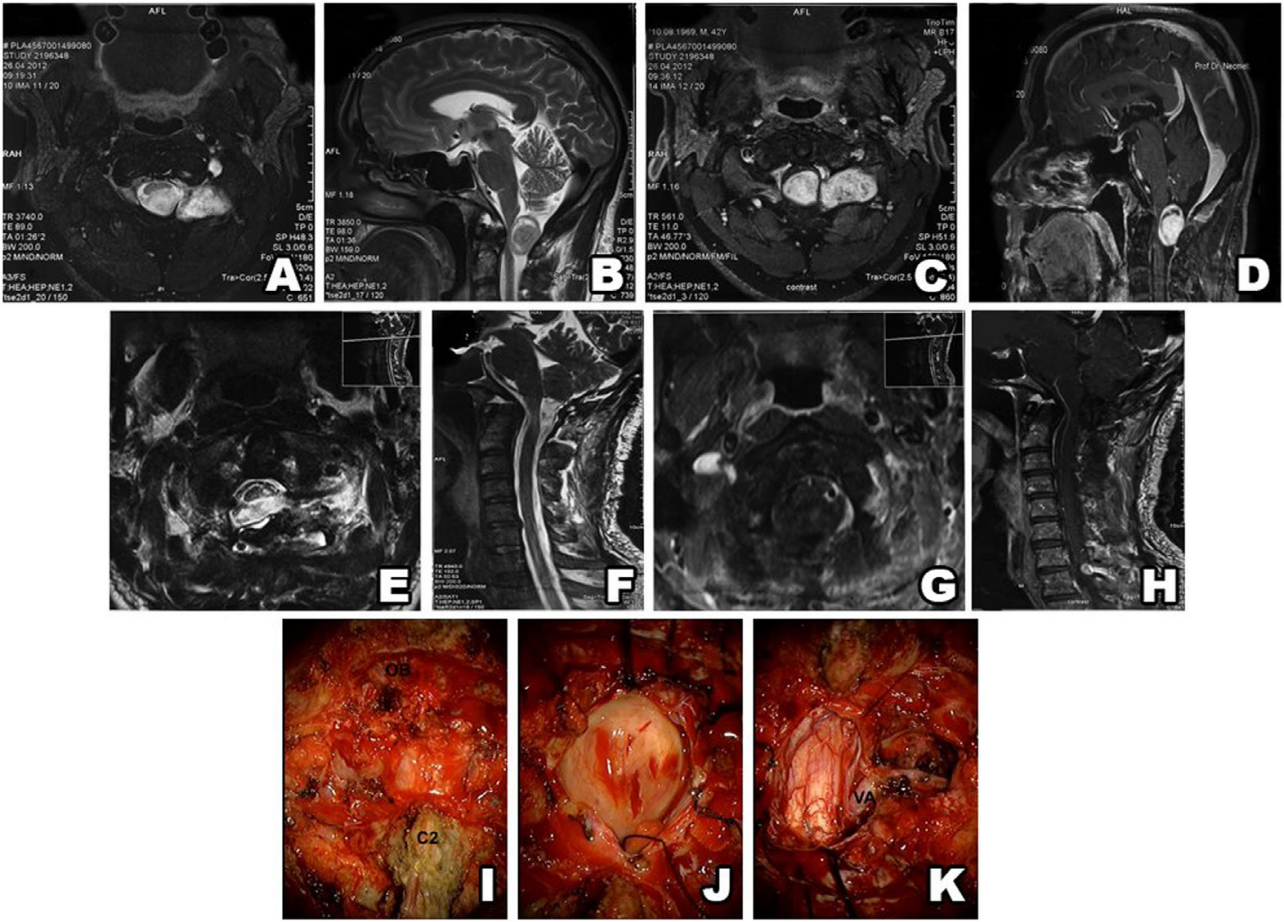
Type III schwannoma (intradural dumbbell-shaped tumor within the spinal canal and extending to the extraforaminal region). Preoperative MRI shows a tumor with extraforaminal extension (A–D). Postsurgery MRI confirms gross total resection, without hematoma or recurrence (E–H). Intraoperative view of a schwannoma extending beyond the spinal canal (I) (OB, occipital bone; C2, second cervical vertebra). Intradural part of a schwannoma (J). Postoperative view of the surgical field shows no tumor residue (K) (VA, vertebral artery).
